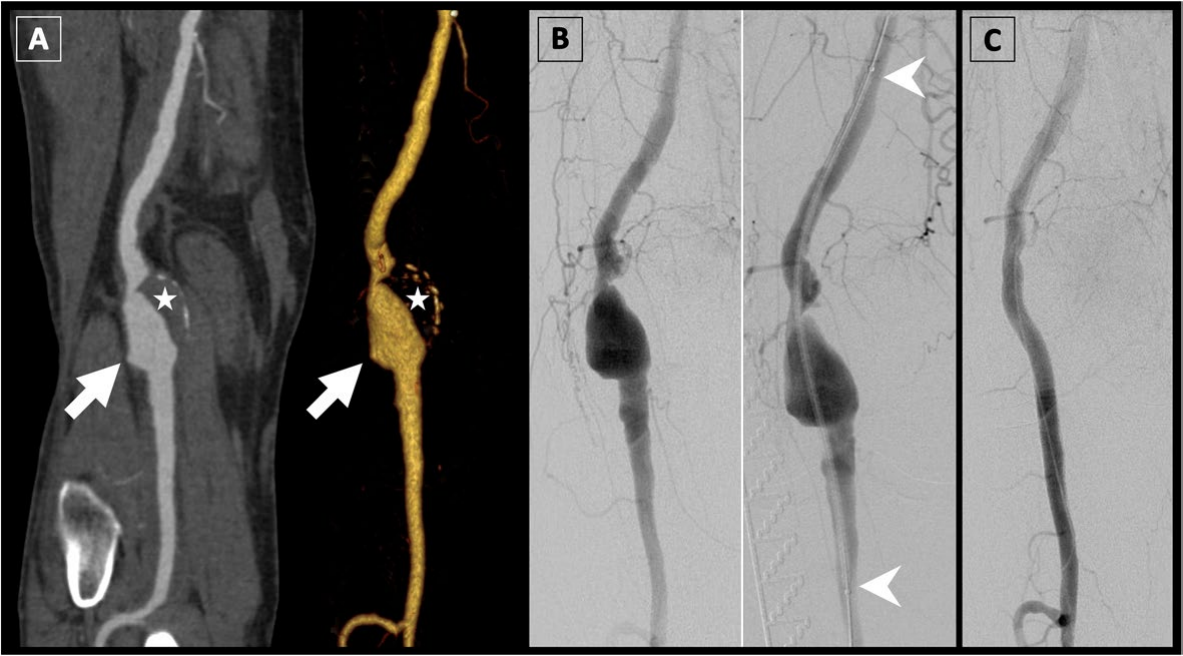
Viabahn endoprosthesis for popliteal aneurysm repair. A 73-year-old man was referred for (A) CT angiography, which revealed a popliteal aneurysm (arrows) with substantial lumen thrombus (stars). B Stent graft repair was performed using a Viabahn endoprosthesis (proximal and distal ends of the stent graft indicated by arrowheads). C Final target site angiography confirmed successful exclusion of the aneurysm and preservation of blood flow
